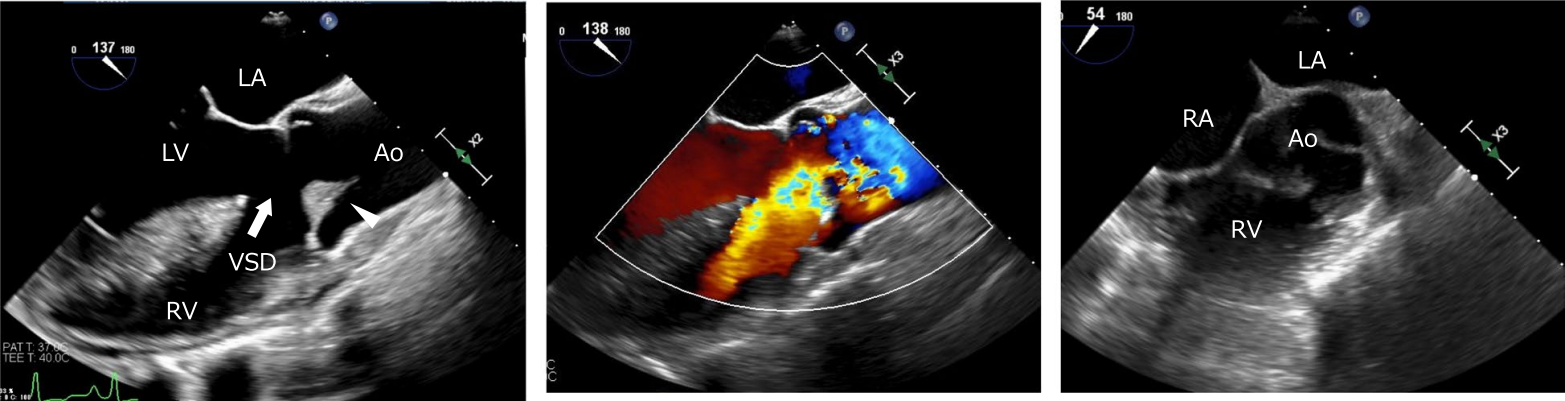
Transesophageal echocardiography revealed a 14 × 7 mm mobile vegetation attached to the right coronary cusp (arrowhead). Moderate aortic regurgitation was detected using transesophageal color Doppler imaging. The right ventricular outflow tract was not detected. Ao, aorta; LA, left atrium; LV, left ventricle; RV, right ventricle; VSD, ventricular septal defect
